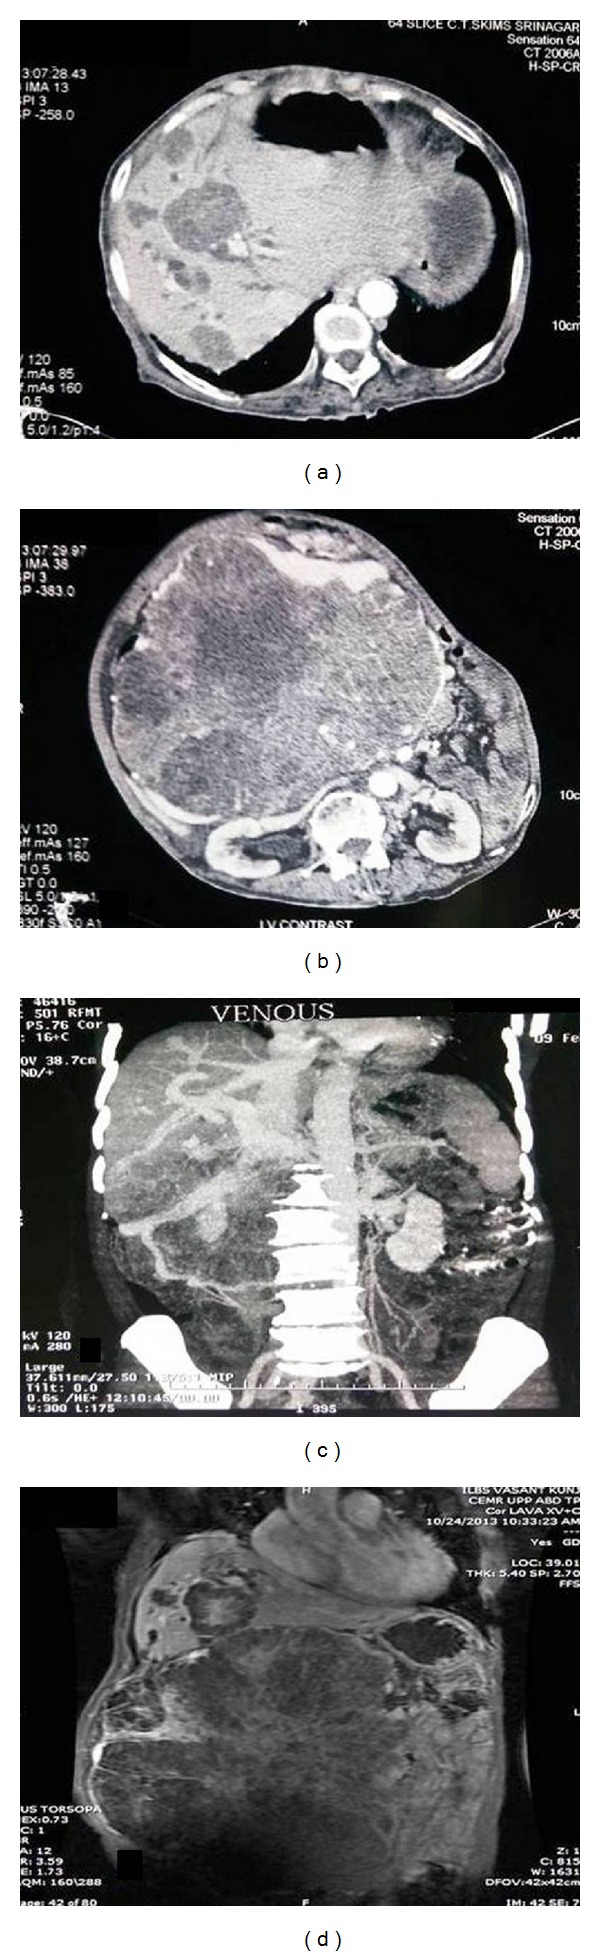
The computed tomographic ((a) to (c)) and magnetic resonance (d) imaging of the abdomen showing features of multiple metastatic lesions in the liver, predominantly in the right lobe (a); 26 cm large heterogenous retroperitoneal mass with areas of necrosis, breakdown, and calcifications involving almost the whole of the abdominal cavity producing mass effect (b); large venovenous communication between the tumor and the major venous channels on venography (c); Large lobulated heterogenous mass with internal septations and surrounding liver metastases extending from the upper to the lower part of the abdomen showing mass effect.
